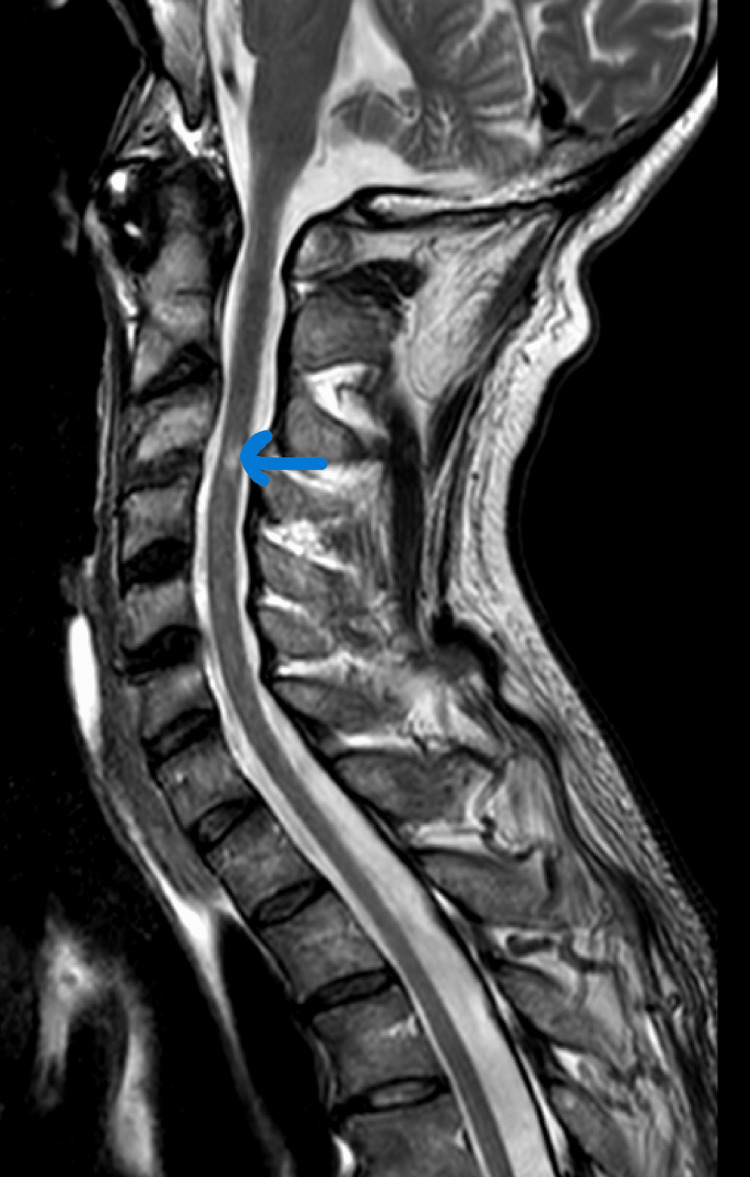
Follow-up sagittal T2-weighted MRI at four months The image demonstrates the complete resolution of the pre-vertebral and epidural abscesses. The C3/C4 disc signal has normalized. A persistent, regressive intramedullary T2 hypersignal (blue arrow) is visible, consistent with chronic compressive myelopathy.
